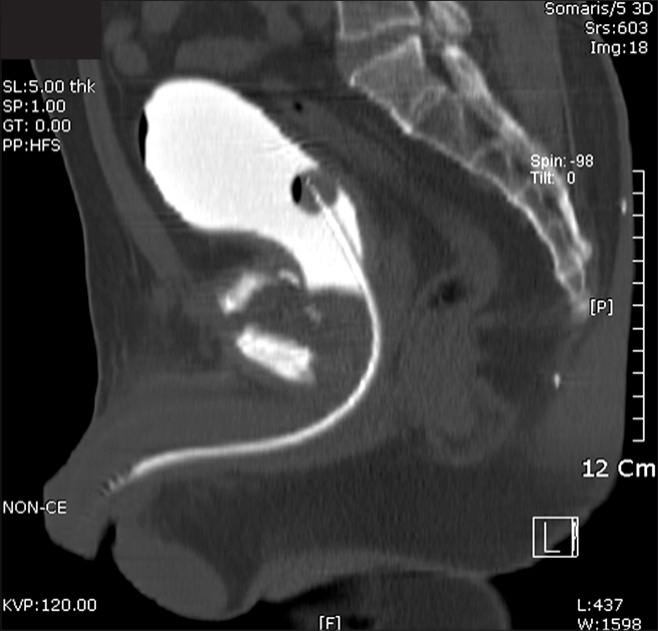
CT cystogram shows no contrast connections from the bladder to the abscess of the pubis and thigh.
